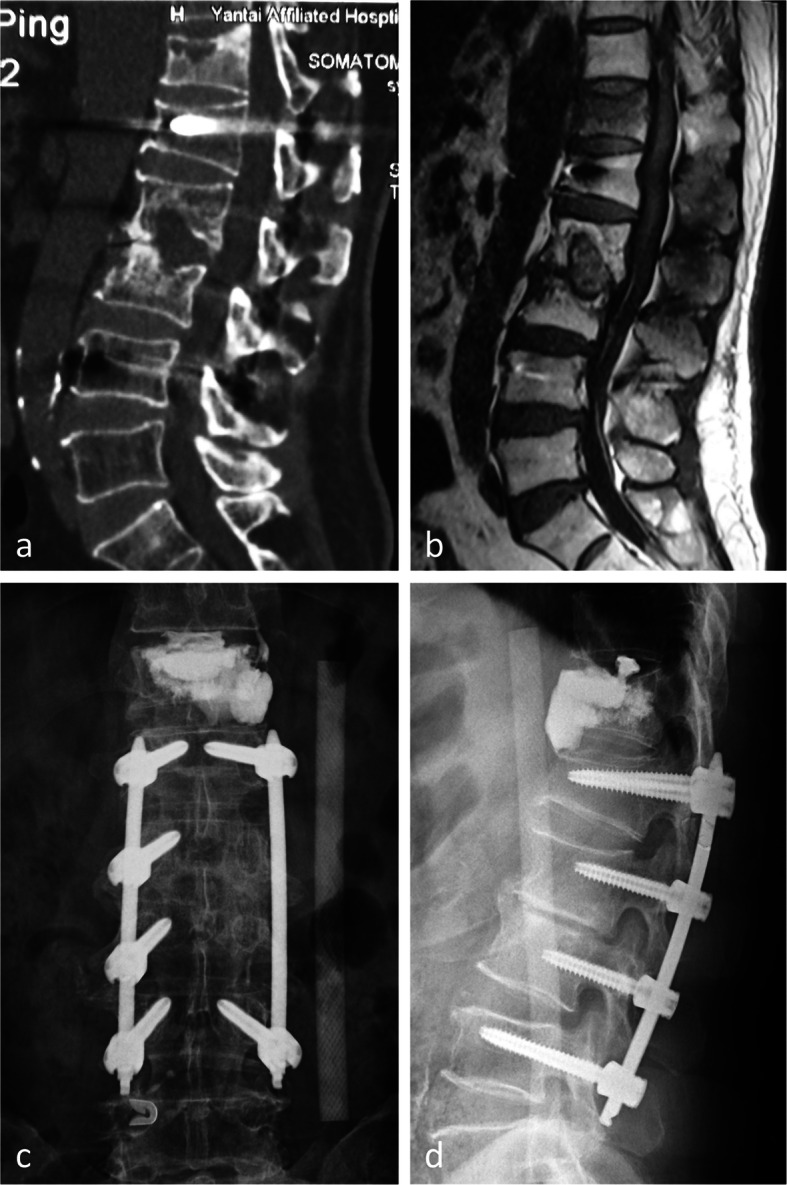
A 60-year-old man suffered from back pain following a fall at 6 months after STB surgery. a, b The CT and MRI showed adjacent segment compression fracture. c, d Anteroposterior and lateral radiograph after percutaneous kyphoplasty
